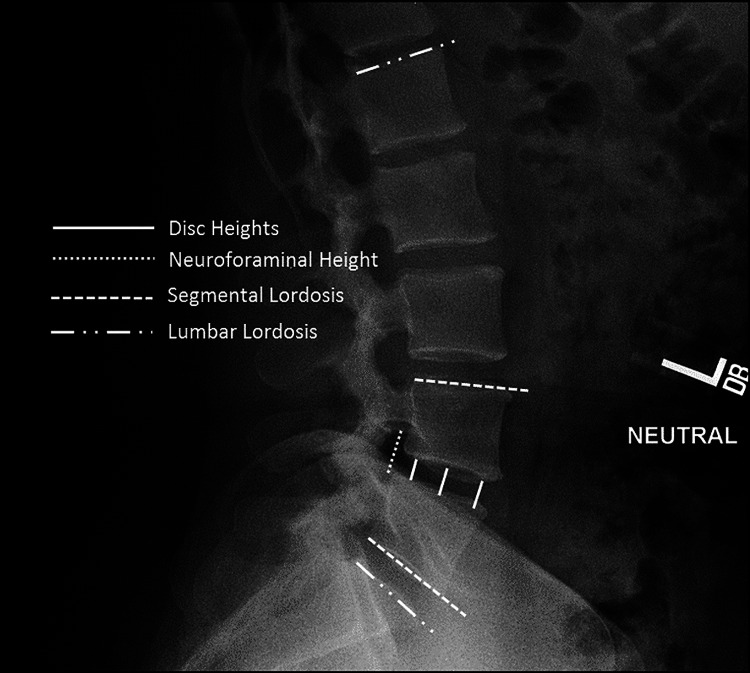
Standing lateral lumbar spine radiograph with superimposed lines displaying the measurements evaluated in this study Measurements included disc heights, neuroforaminal height, segmental lordosis, and lumbar lordosis
